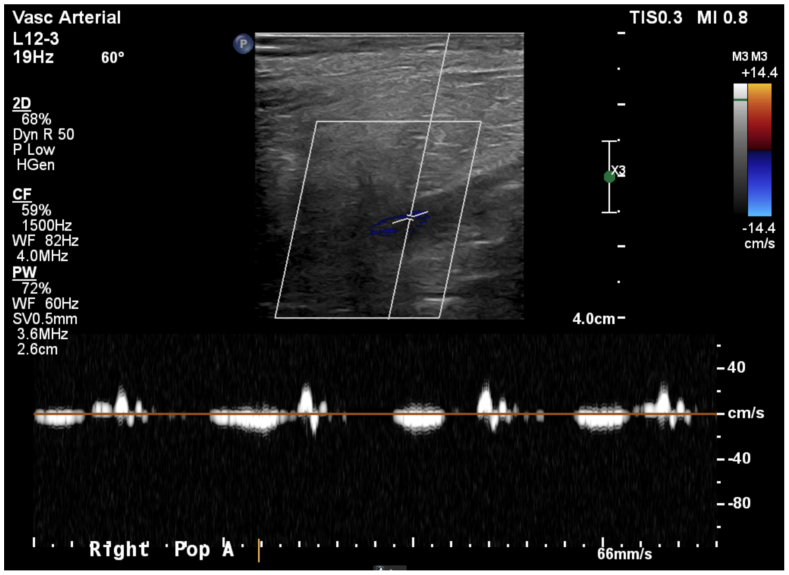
Duplex ultrasound demonstrating presteal physiology in distal superficial femoral artery (SFA) and popliteal artery.
